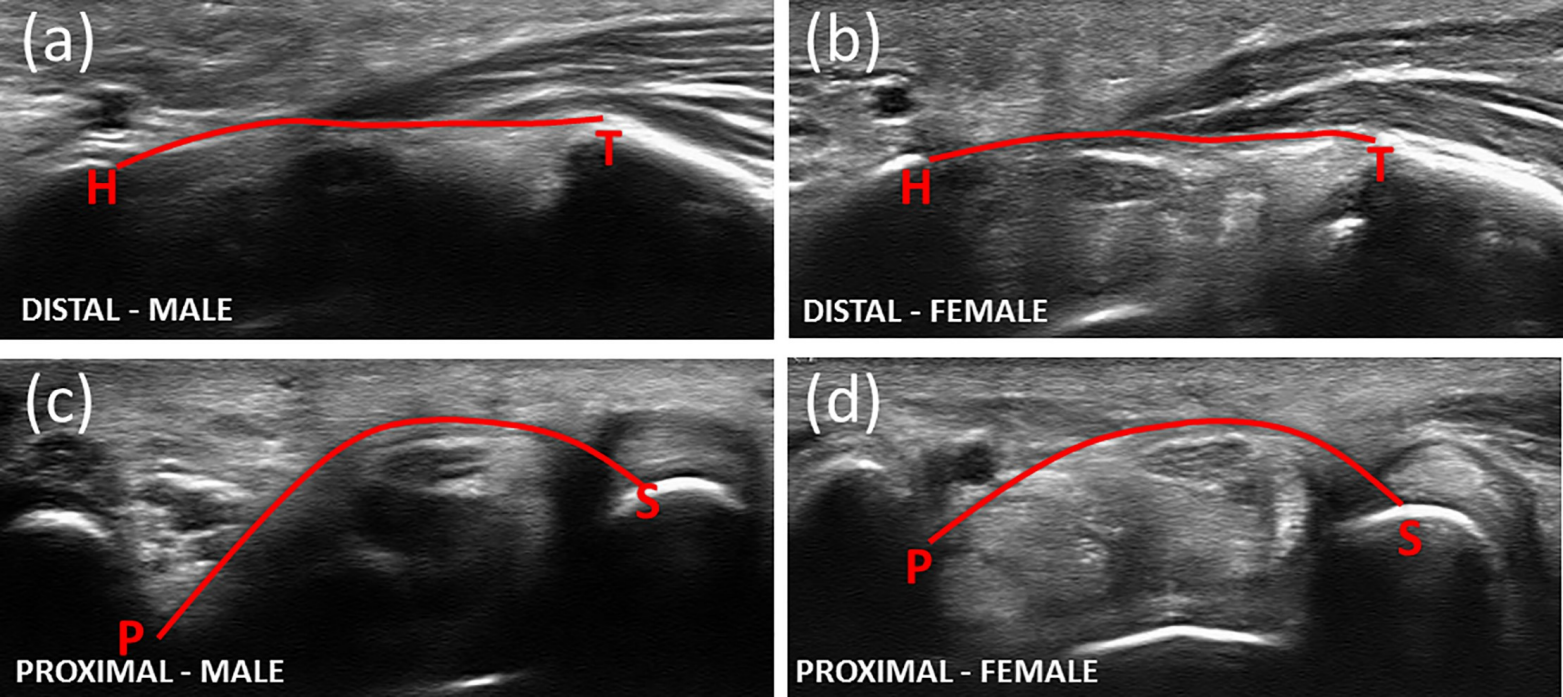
Ultrasound images at each tunnel level (distal and proximal) for a representative male and female subject. The images reflect the selection made in the custom LabVIEW code for the traced TCL (solid line) and the landmark of interest, specifically the hook of hamate (H), ridge of the trapezium (T), pisiform (P), and scaphoid (S).
